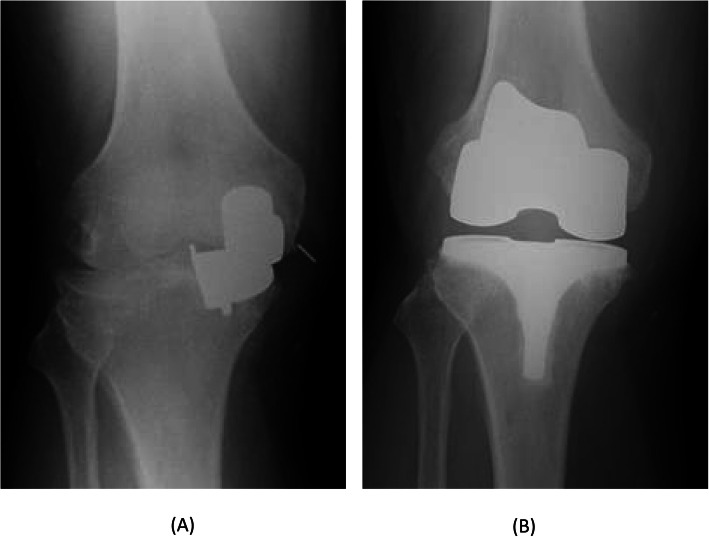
Radiographs 5 months after the additional surgery shows bearing re-dislocation (a). Radiographs after the revision to total knee arthroplasty (b)
